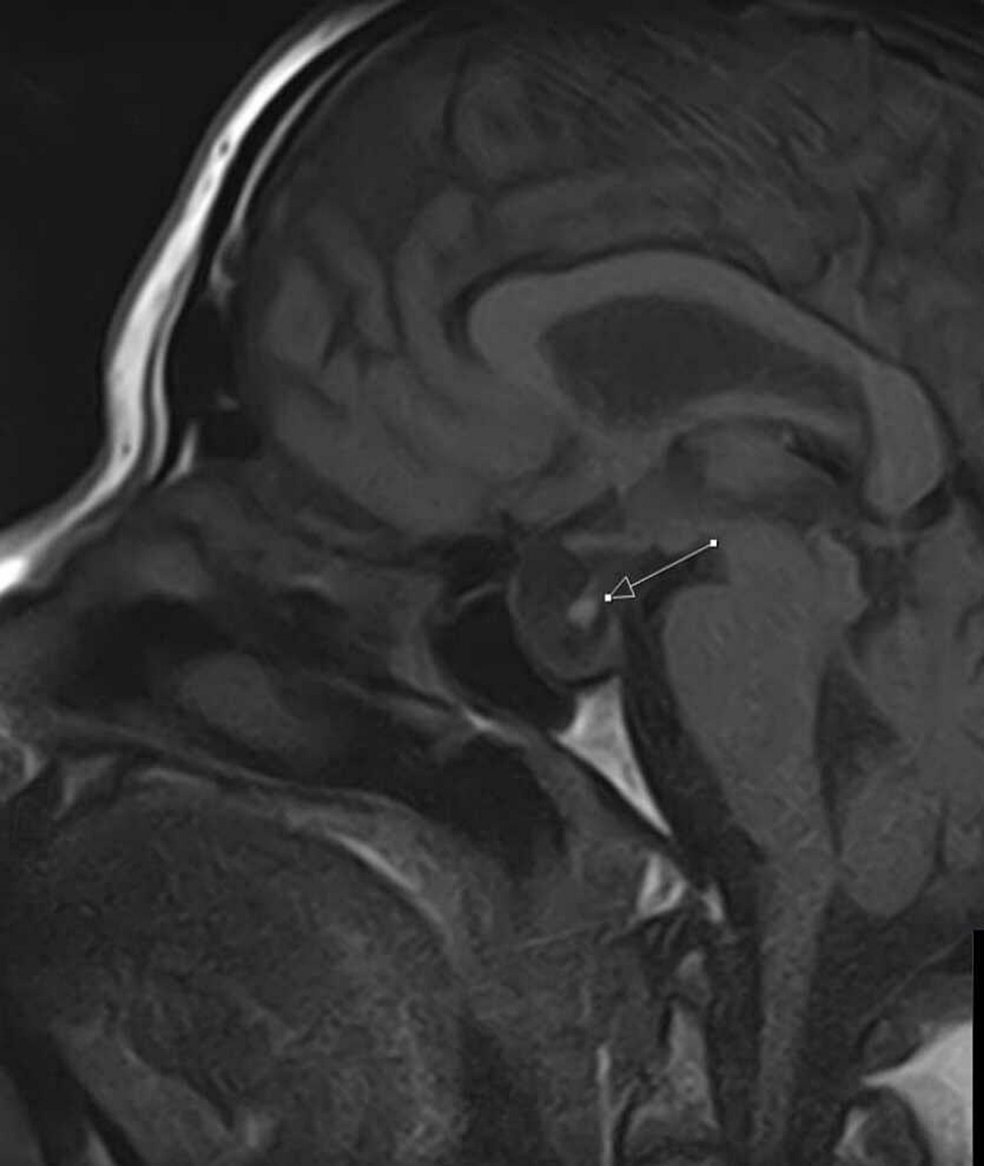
MRI head post transsphenoidal surgery shows a very small residual tumor
